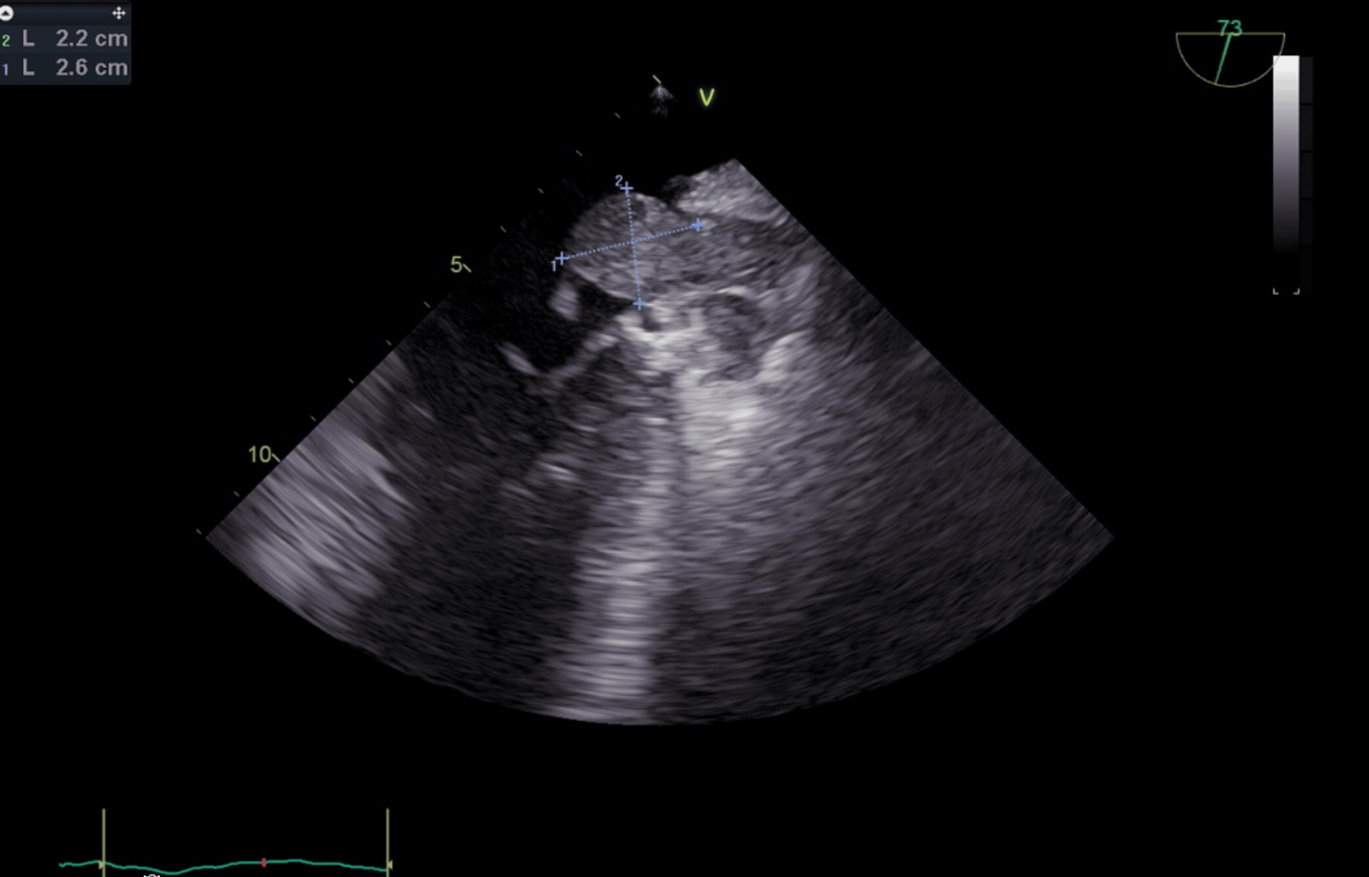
Transesophageal echocardiogram showing a 2.2 cm × 2.6 cm mass inside the left atrium indicated by the blue dashed lines
